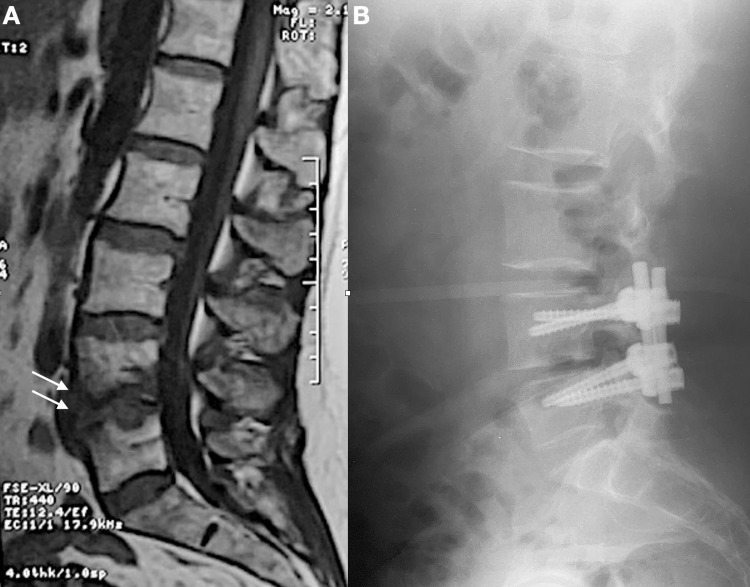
A. Preoperative sagittal T1-weighted image of the lumbar spine, showing L4-L5 spondylodiskitis (arrows); B. Postoperative lateral radiograph after debridement, decompression, and posterior instrumentation
